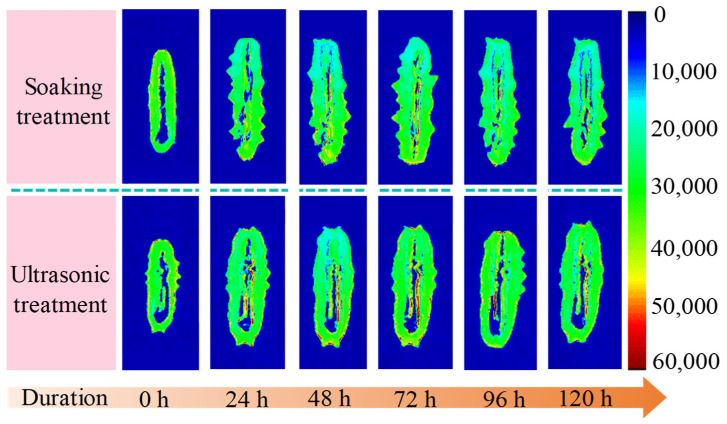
Water distribution in semi-dry salted *Apostichopus japonicus* treated with ultrasonic and traditional soaking methods.
